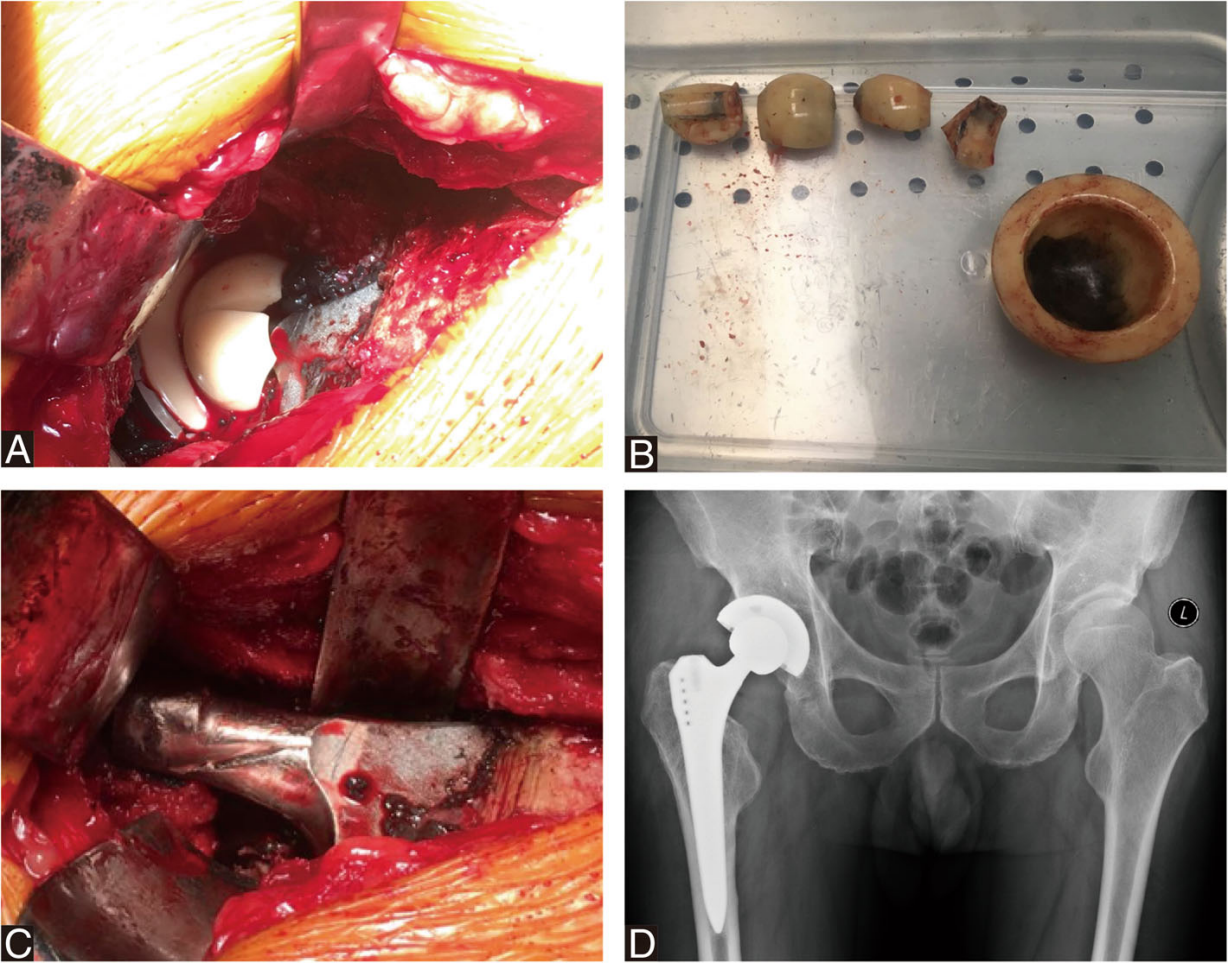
Revision MoP total hip arthroplasty for ceramic head breakage. a-b Intraoperative photograph demonstrating ceramic head breakage and (c) severe wear of the taper and neck of femoral stem. d Postoperative anteroposterior radiograph after revision with metal head and polyethylene liner
